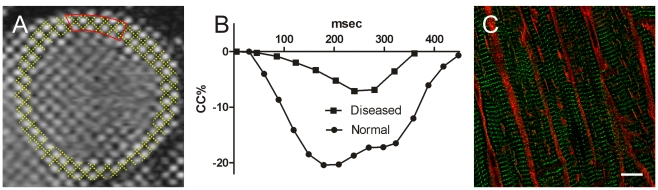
Panel A shows tagged MRI short axis image through the middle of a failing heart in end diastole. Tracked grids are shown as yellow overlay with sampled region marked in red. Panel B shows circumferential shortening (CC%) of the region indicated in the panel A compared to a similar region in normal human heart. Panel C shows RyRs (green) and WGA (red) labeling of diseased myocytes from the region shown in panel A. Image is z projections of 10 slices with z depth of 2.5 µm from deconvolved image stack. Scale bar is 10 µm.
